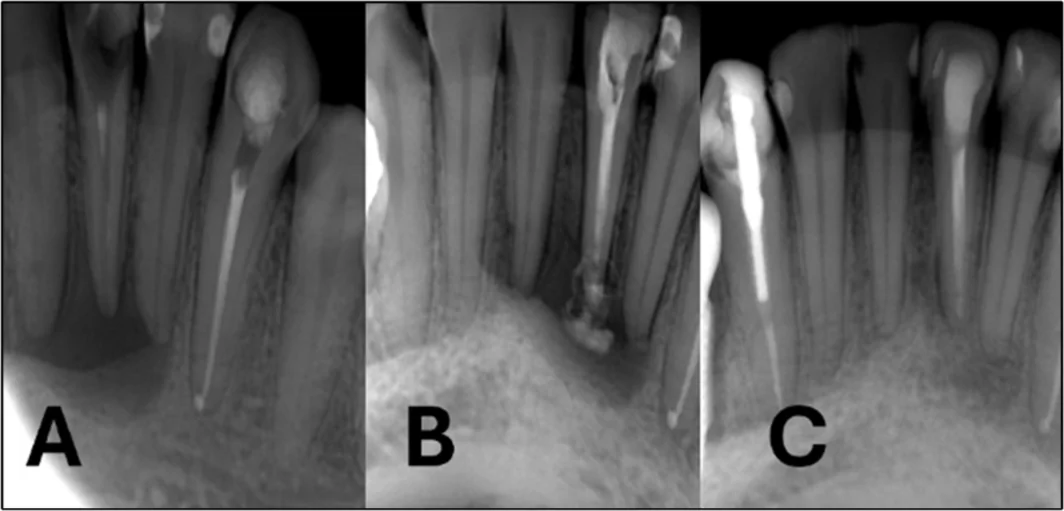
Case 1. (A) Preoperative periapical radiograph showing inadequate previous root canal treatment and a large periapical radiolucency associated with tooth #24. (B) Immediate postoperative radiograph following nonsurgical retreatment using a 5‐mm CC apical plug with apical extrusion of CC. (C) Four‐year follow‐up periapical radiograph showing that the previously observed periapical radiolucency was no longer evident and that the extruded CC was no longer distinguishable radiographically.
